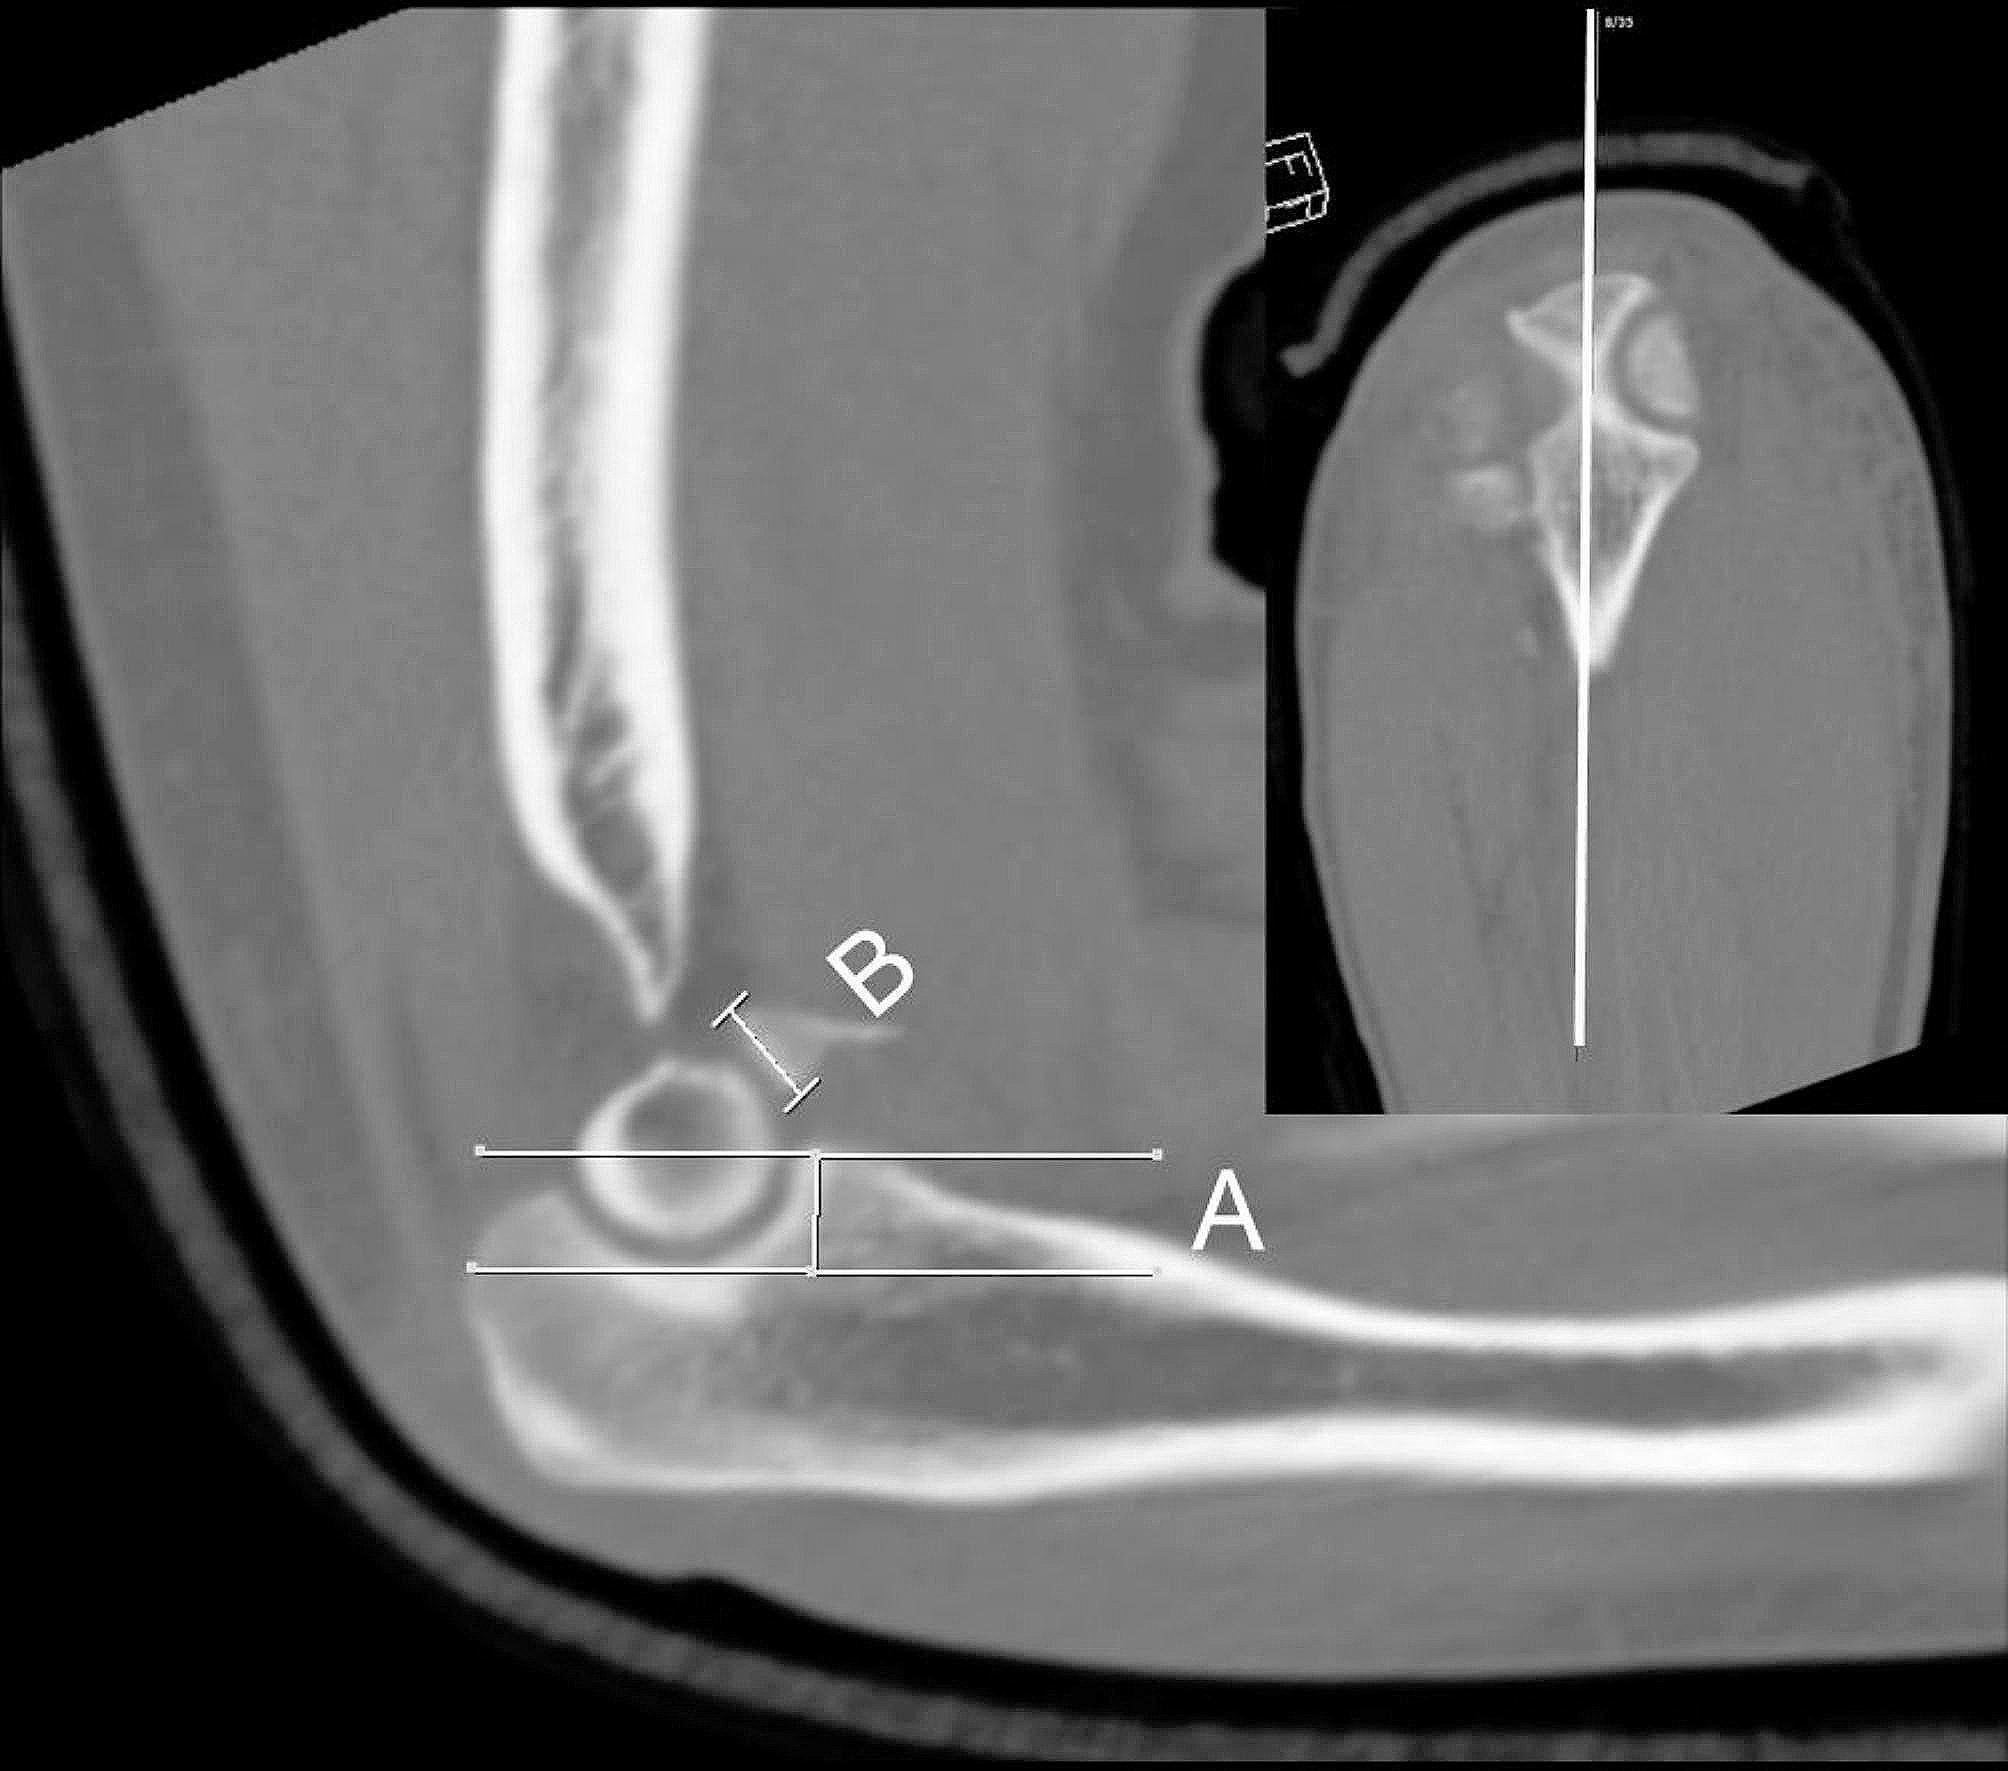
Preoperative evaluation of height loss of the coronoid process using computed tomography (CT). Height loss of the coronoid process is evaluated using a preoperative CT study. After the midline portion at the coronal plane is identified, it is used as a reference to confirm the mid-sagittal plane of the coronoid process. In the mid-sagittal plane, the height loss of the coronoid process is evaluated by measuring the ratio of the height of the fractured fragment (b) to the total expected coronoid process height (a + b)
